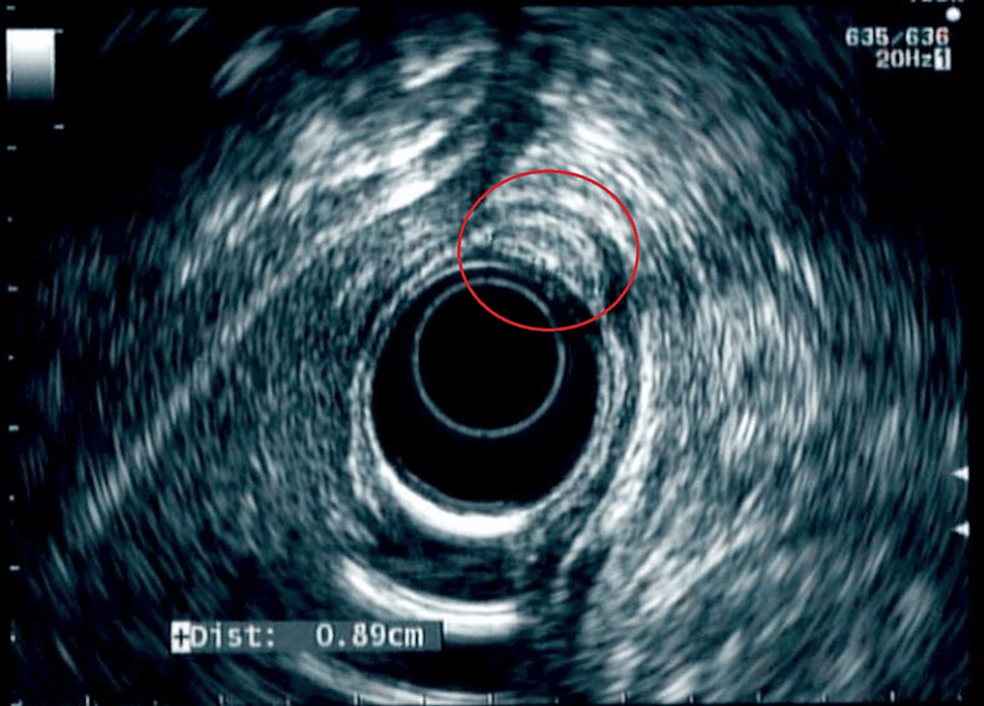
EUS showing nodule arising from muscularis mucosa, hypoechoic with heterogeneous areas (red circle) EUS, endoscopic ultrasound
